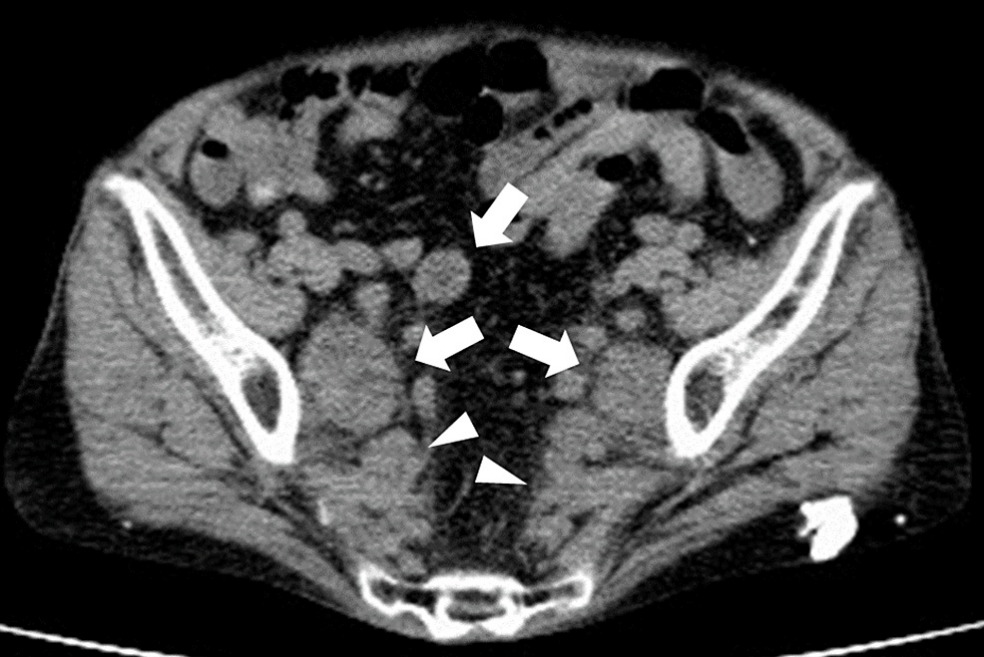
CT CT indicates multiple swollen lymph nodes (arrows) and a thickened retroperitoneum (arrowheads). CT: computed tomography
